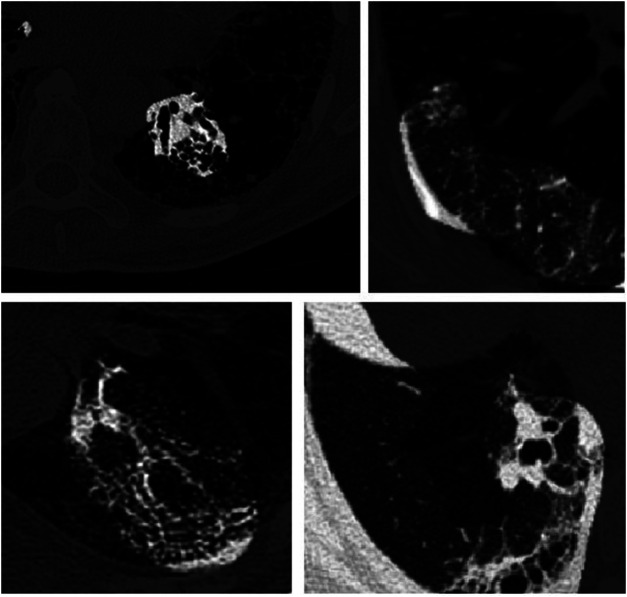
Example critical factors (highlighted as brighter regions) in example CT images of pulmonary fibrosis patients as identified by GSInquire (Lin et al., 2019). It can be observed that Fibrosis-Net is capable of leveraging clinically relevant visual indicators such as the presence and geographic extent of honeycombing in the lungs as presented in the CT images to make FVC predictions.
